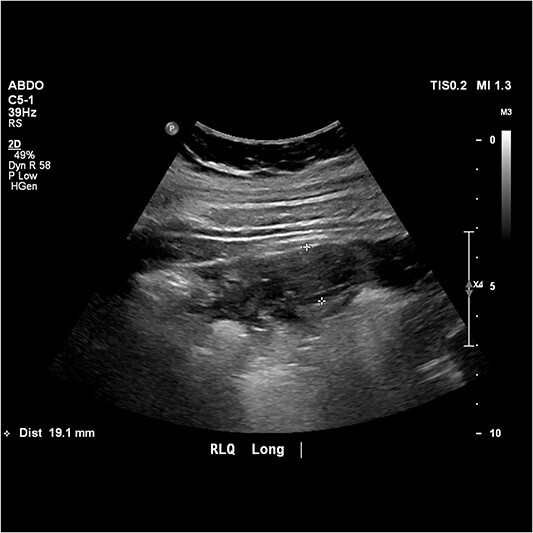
Image from abdominal ultrasound demonstrating hyperaemic, tubular structure measuring 19.1 mm in width with echogenic fat.
